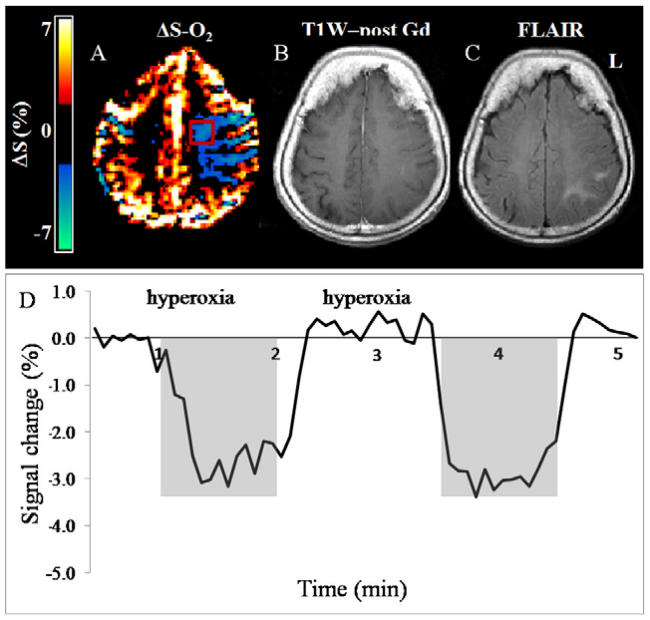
Negative ΔS-O2 response. Representative results obtained from a patient with recurrent GB (patient number 4), showing negative ΔS-O2 response during hyperoxic challenge observed distant from the tumor area. (A) ΔS-O_2_ map; (B) T_1_WI post contrast agent injection and (C) fluid attenuation inversion recovery (FLAIR) image. (D) The mean time courses of the signal intensity change (%) during hyperoxic challenge obtained from the area with negative response (VOI is marked by red rectangle on Figure 4A).
